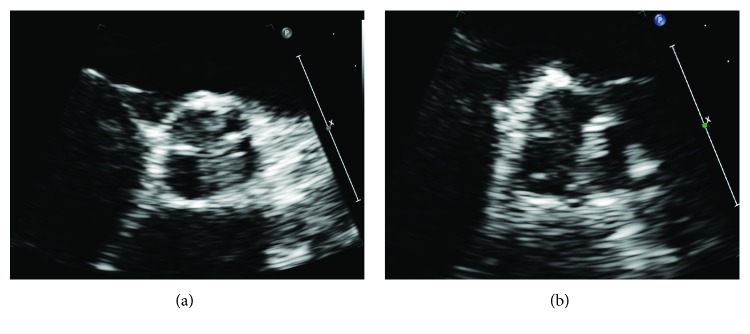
Echocardiogram demonstrating a vegetation proximal to the left aortic cusp (a) and the vegetation mobilizing toward the left coronary artery origination anastomosis upon valve opening (b).
